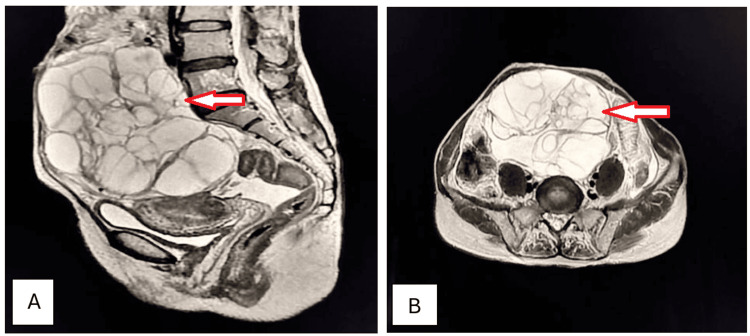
Mucinous cystadenoma of the right ovary (arrows). Sagittal (A) and axial (B) T2W MRI imaging shows a well-circumscribed, large, multiloculated, cystic mass in the pelvis with numerous internal septations, appearing predominantly hyperintense but of variable signal intensity because of the mucin content; the mass is overlying the uterus and causing a mass effect on the urinary bladder and uterus and displacing them inferiorly, which is consistent with a mucinous cystadenoma of the ovary. T2W: T2-weighted
